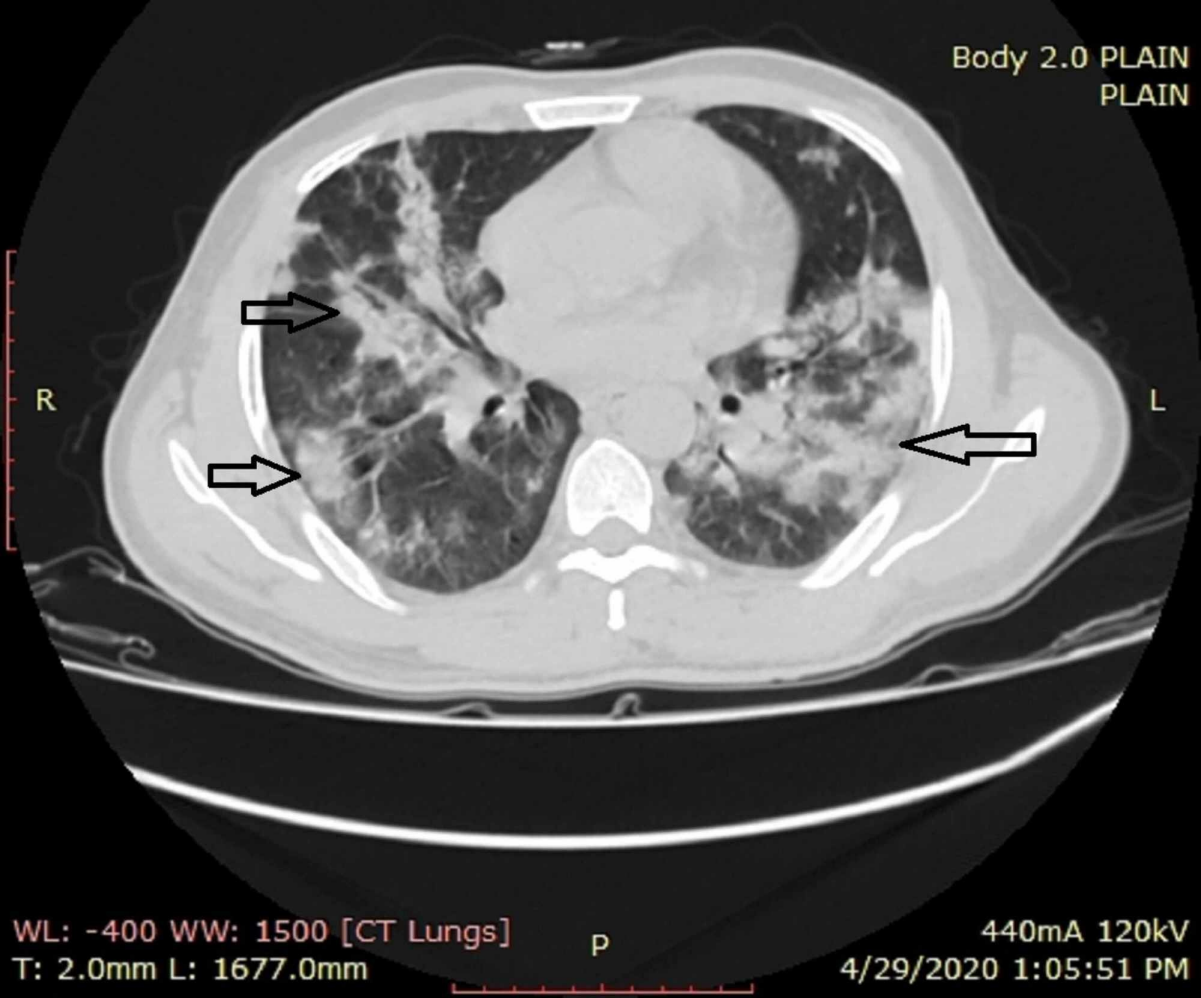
A female patient with dropping oxygen saturation CT shows multiple bilaterally predominant areas of consolidations with interspersed areas of ground-glass opacities in peripheral as well as central distribution (arrows) CT: computed tomography
